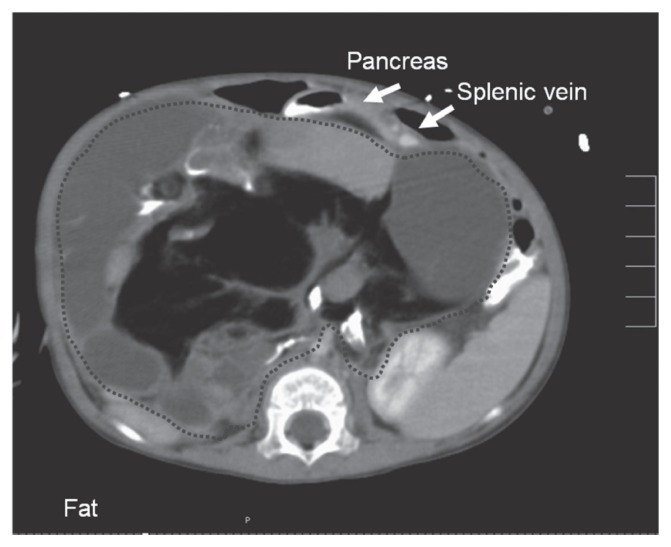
The same tumor as in Figure 1 in a transversal section. Pancreas and splenic vein are displaced towards ventral. (MRI, T1, transversal).
